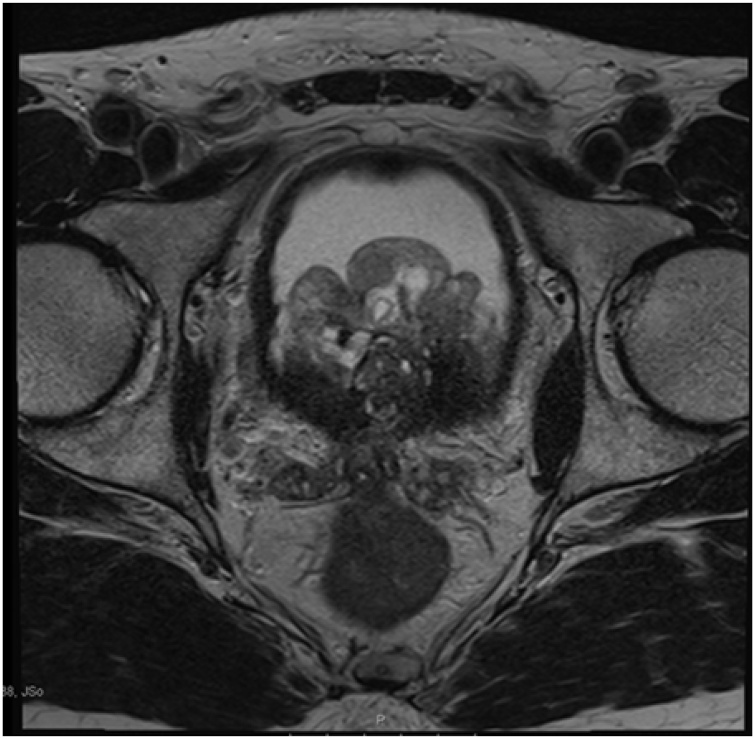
T2-weighted MRI scan (axial view) shows the regrowth of the prostate gland into 131 ml protruding to the bladder base after only one year of TURP.
